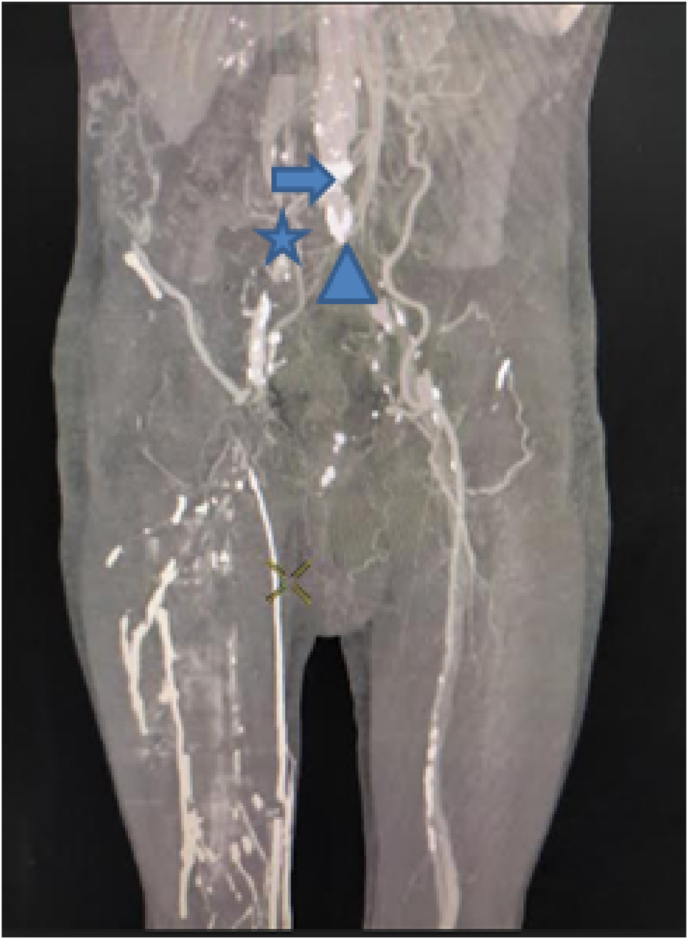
CT Angiography in coronal view showing a massive thrombus in the aortoiliac region Arrow: aorta star: right common iliac artery triangle: left common iliac artery.
